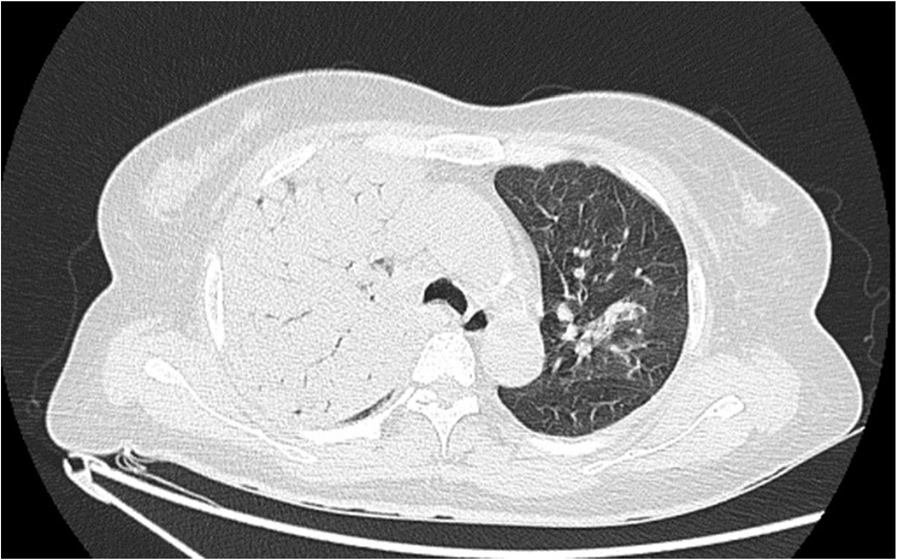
Chest computed tomography (CT) scan of a 60-year-old woman with severe pneumonia demonstrated large areas of consolidation in the right lung, ill-defined consolidation in the left lung, partial tracheobronchial stenosis in both lungs, and polyglandular mediastinal lymphadenopathy
